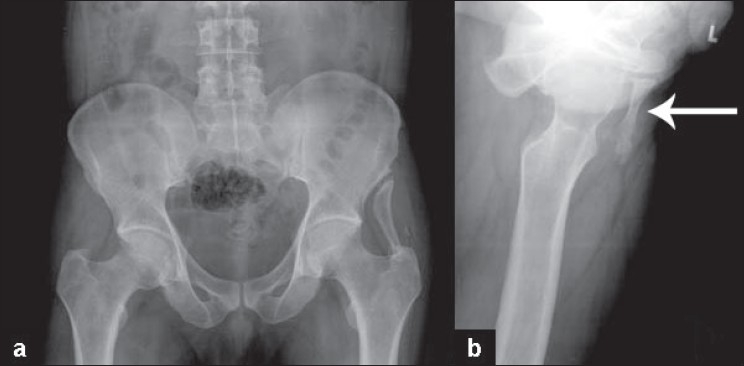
(a) Plain radiograph of the pelvis (anteroposterior view) depicting a finger-like bone projecting on the left iliac wing with pseudo-articulation with the ilium. (b) Lateral radiograph of the left hip showing a finger-like bone projecting (white arrow) on the left iliac wing.
